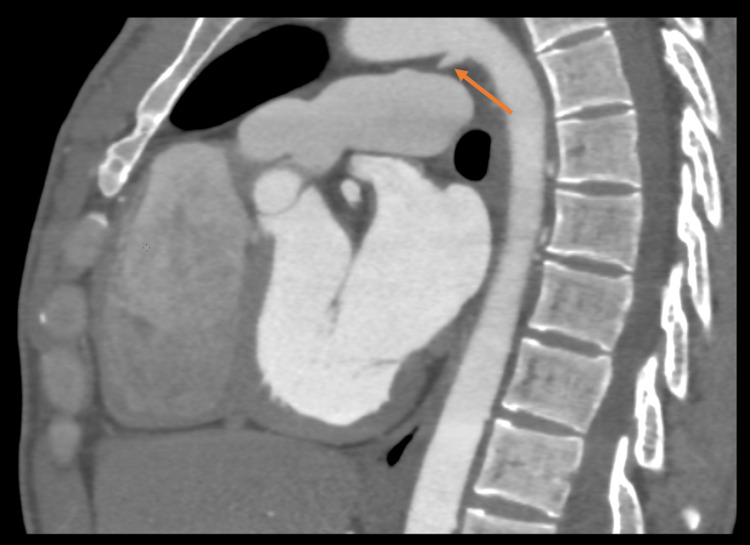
Sagittal image of coronary CT angiogram showing incidental conical-shaped patent ductus PDA (arrow) PDA: patent ductus arteriosus
